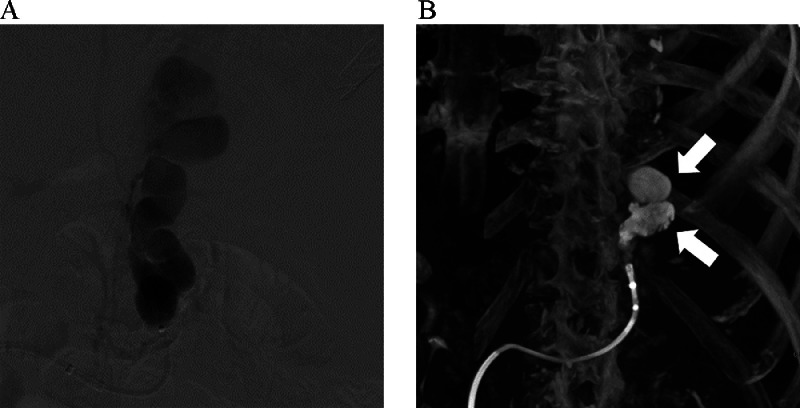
A 71-year-old patient with gastric varices (case 2). (A) After inserting a balloon catheter into the gastrorenal shunt, 5% ethanolamine oleate iopamidol was injected under balloon occlusion. (B) Three-dimensional image derived from cone-beam computed tomography without contrast media showed that injected *n*-butyl cyanoacrylate (arrow) formed a cluster in the gastrorenal shunt under flow control.
